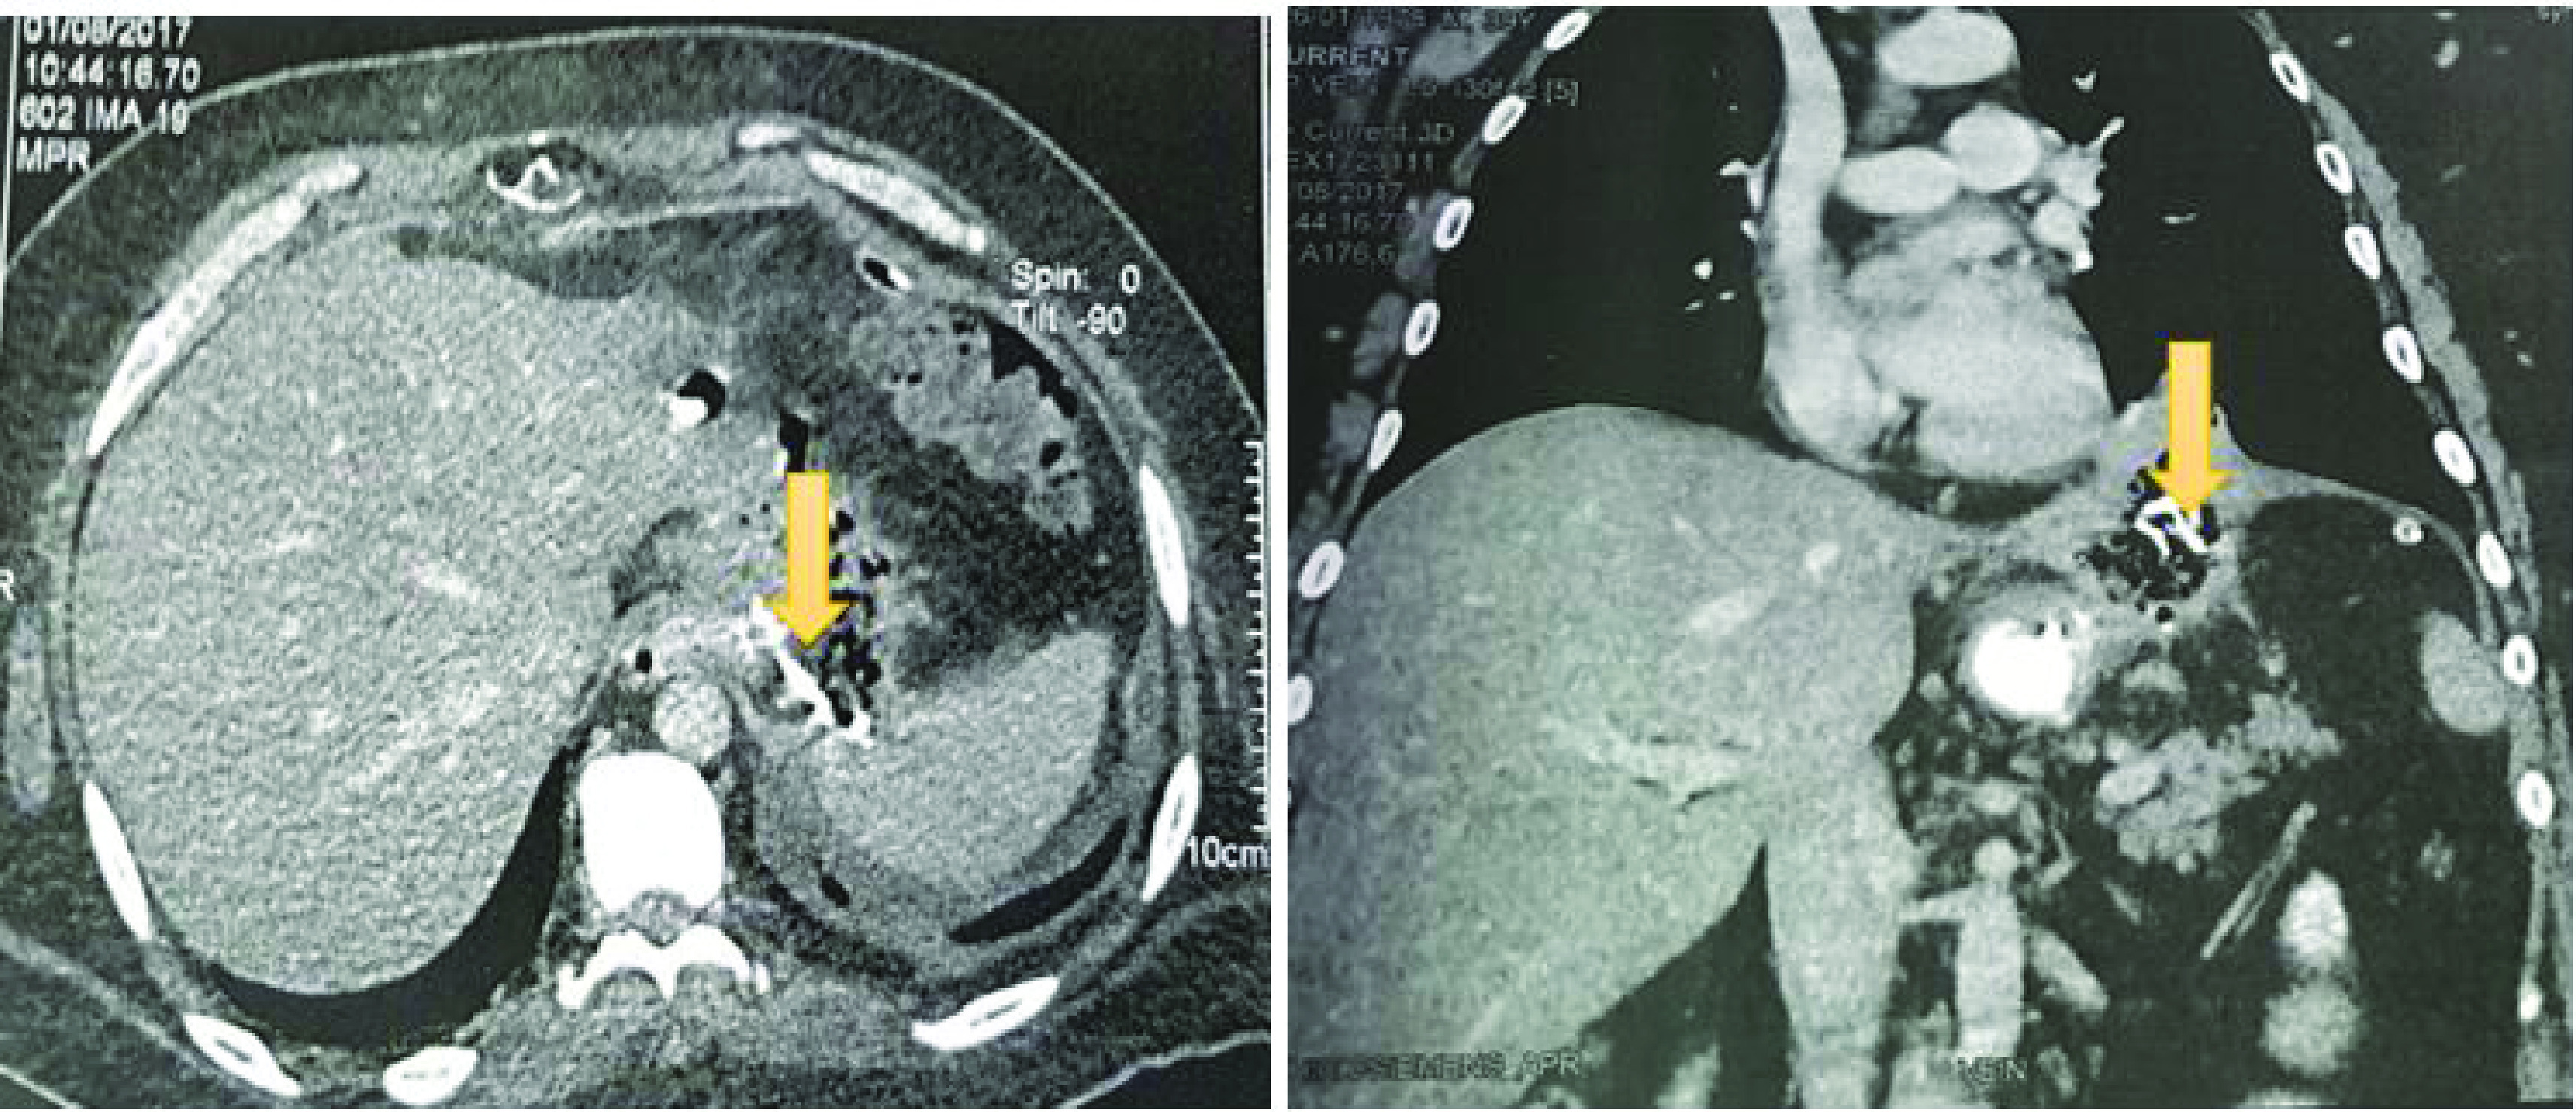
CT scan axial view showing a slight decrease in the size of the subphrenic collection with a persistent fistula and a spongiform formation with opaque serpiginous structures (yellow arrow) typical of a textiloma.
